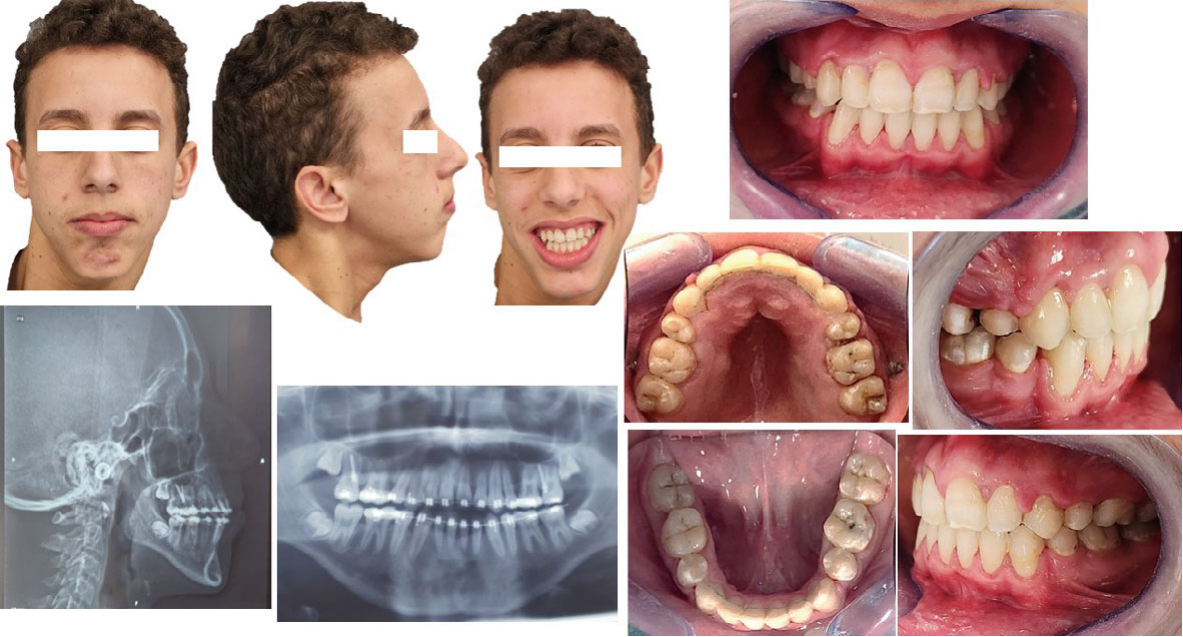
Post treatment photos and radiographs.
